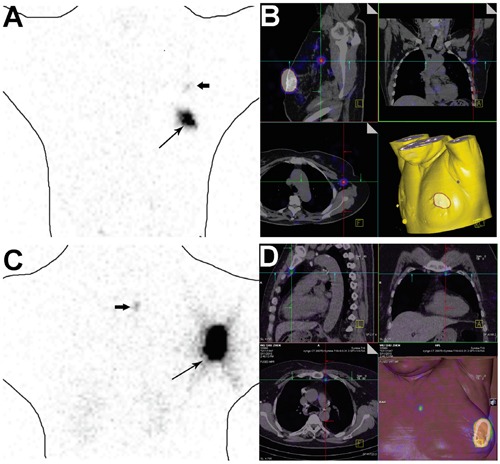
A-B. A 54-year old female with an infiltrating ductal carcinoma of the left breast. A sentinel lymph was localized in left axillary on sentinel planar lymphoscintigraphy (A) and SPECT/CT (B). C-D. A 37-year-old female with an infiltrating ductal carcinoma of the left breast. Sentinel planar lymphoscintigraphy (C) and SPECT/CT (D) identified an internal mammary node as the SLN.
